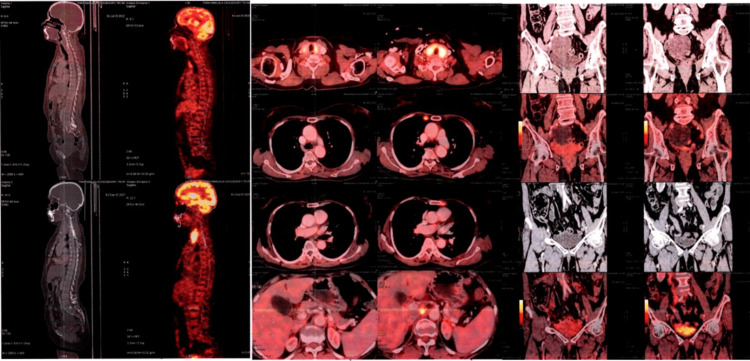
The PET/CT shows a complete response maintained on rucaparib at the 11th-month follow-up PET/CT: Positron emission tomography-computed tomography
